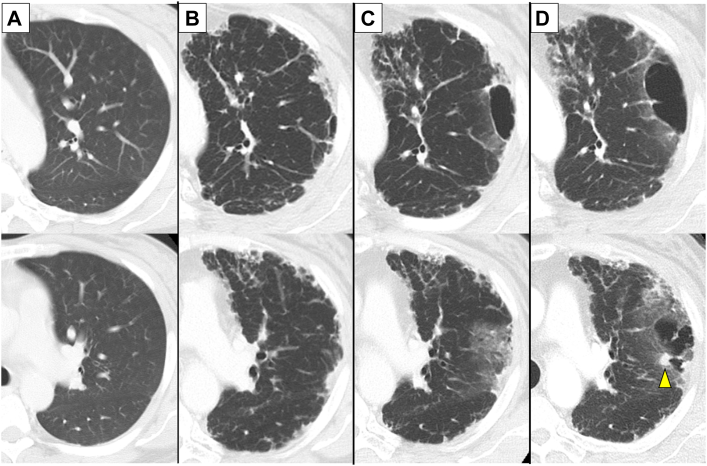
Time-course changes on computed tomographic images of the left upper lobe. (A) No significant findings were shown in the recipient’s lung at the time of liver transplantation. (B) A subpleural reticular shadow and consolidation were indicated 9 years after liver transplantation. (C) A pulmonary cyst appeared in the reticular shadow 12 years after transplantation. (D) The cyst had enlarged, and a solid nodule (arrowhead) was observed adjacent to the cyst 12 years and 8 months after transplantation.
